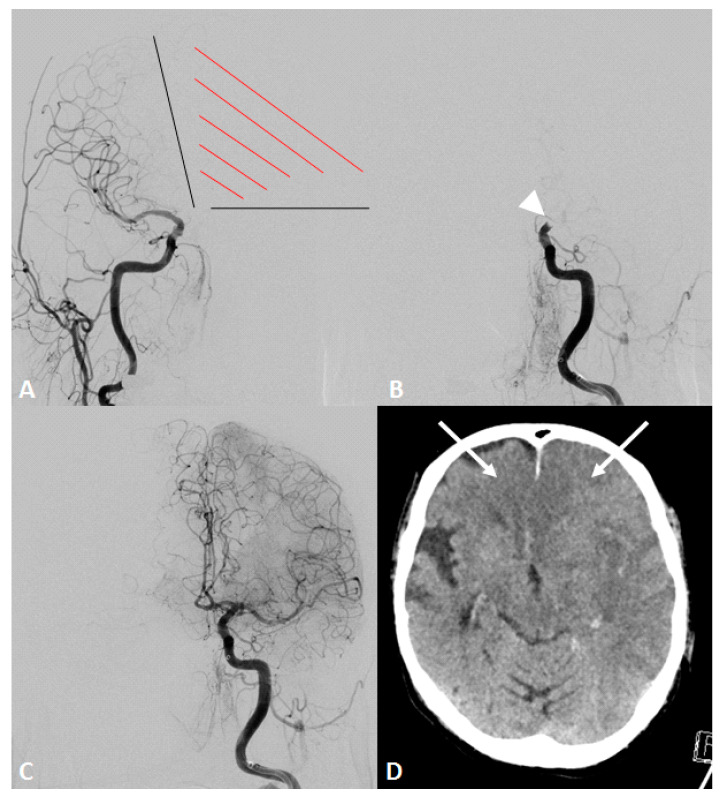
An octogenarian patient presented with an acute onset of right-sided hemiplegia and disturbed consciousness. Initial computed tomography showed an occlusion of the distal internal carotid artery (ICA) on the left side and no detectable infarction (not shown). Intravenous thrombolysis was initiated, and the patient was immediately transferred to the angio suite. (A) Initial angiogram of the contralateral ICA demonstrated an aplasia of the a1 segment and missing collaterals to the affected hemisphere, including both anterior cerebral artery (ACA) territories (red shaded area). (B) Angiogram of the left ICA showing distal ICA occlusion (white arrowhead). (C) Final angiogram after mechanical thrombectomy using two maneuvers with stent-retrieving showed a successful recanalization of the ICA with complete reperfusion of the middle cerebral artery (MCA) and both ACA territories. (D) Computed tomography on the next day demonstrated an expanded cerebral infarction in MCA (Alberta stroke programme early CT score of 0) and both ACA territories (white arrows). The patient died on the same day.
